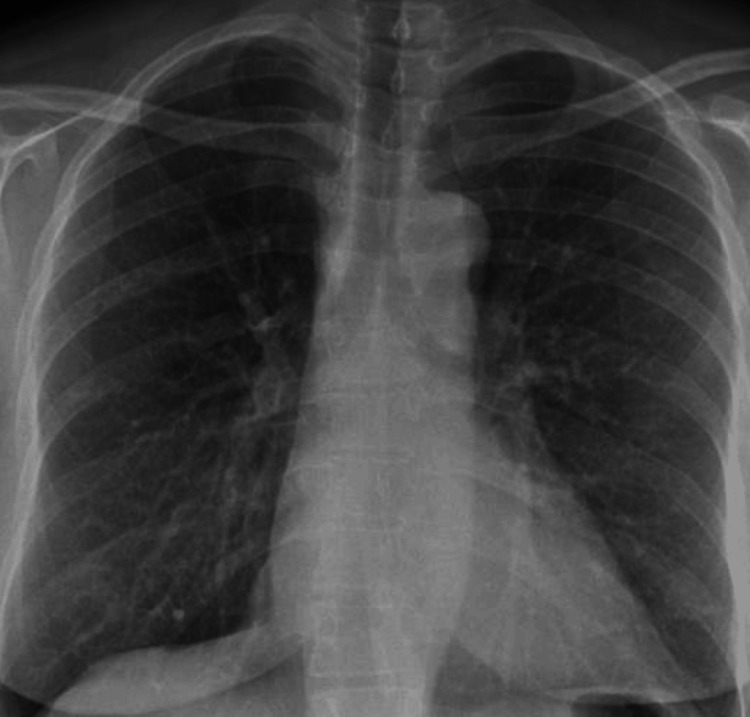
Chest X-ray taken six months after discharge from the hospital. The image shows complete resolution of consolidation as a result of excellent response to steroid therapy.
